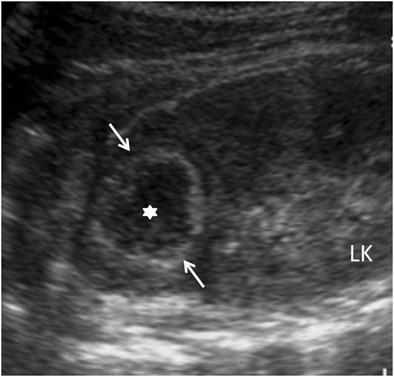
Focal XPN. A 13-year-old boy with left lumbar pain after playing football the day before. Longitudinal sonographic image shows focal hypoechoic mass (*asterisk*) with surrounding hyperechogenic rim (*white arrows*) in upper pole of left kidney
